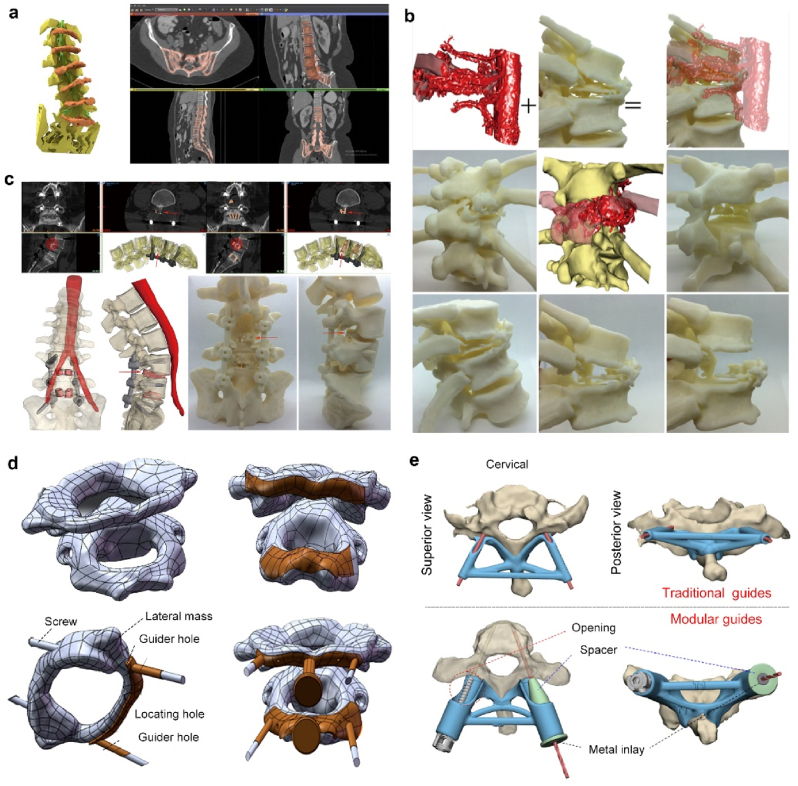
Examples of 3D printed (a–c) anatomical models and (d–e) screw guides in spinal surgery. (a). Screenshot of lumbar spine CT segmentation by computer (left) and the 3D printed spinal anatomical model (right) [206]. (b–c). Examples of 3D printed anatomical models for preoperative planning by (b) depicting the complex vascular distribution of spinal tumors or (c) complex revision surgeries (L4-S1) [208]. (d). Design of the guide template for posterior atlantoaxial pedicle screw implantation [214]. (e). Two types of pedicle screw implantation guides, including traditional personalized drilling guides (above) and innovative modular guides (below) [215].
